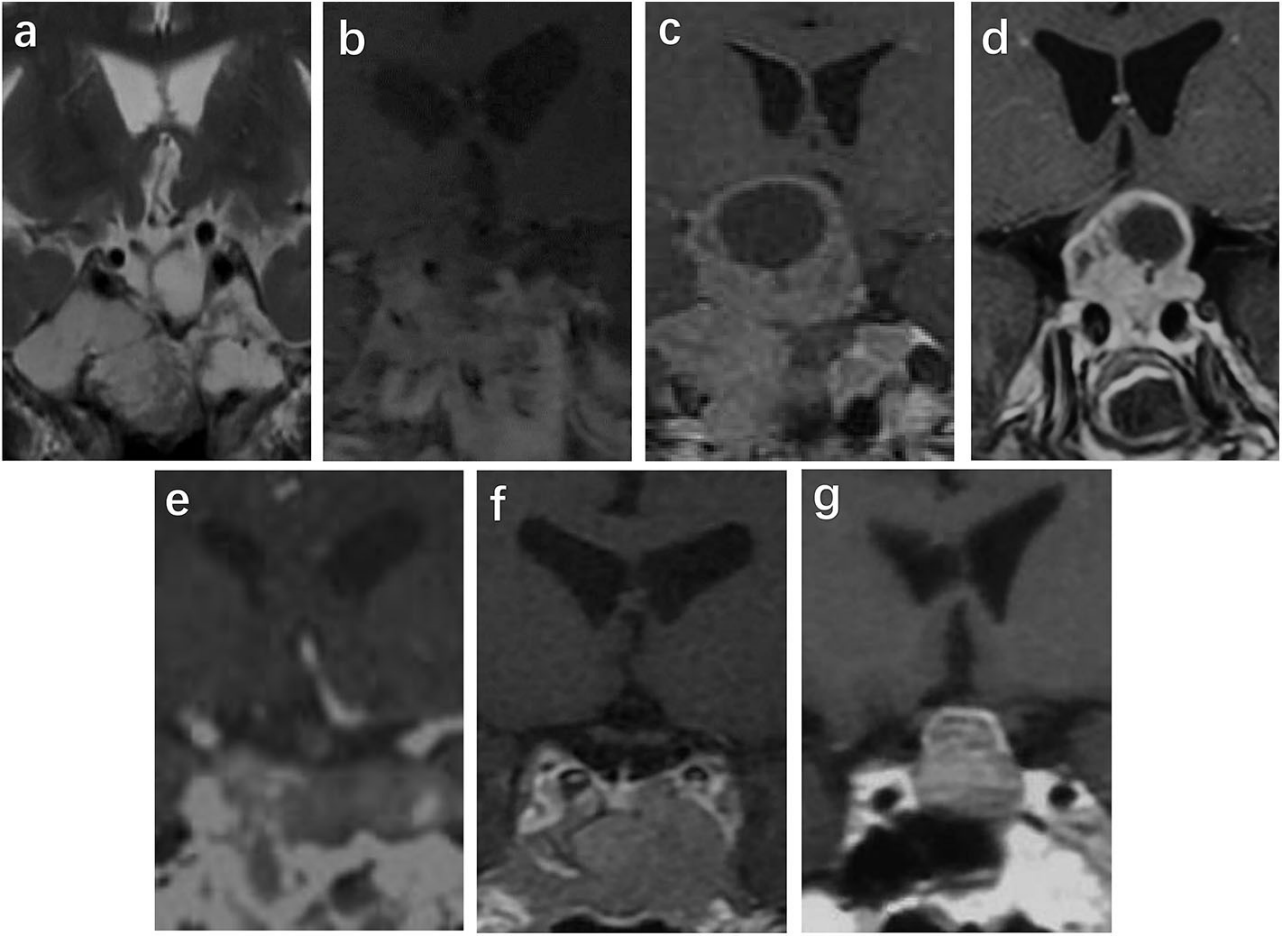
Images at the time of diagnosis of a functional SCA in cases 1–7 (a–g). Sella MRI was performed in cases 1 (a), 2 (b), 3 (c), 4 (d), 6 (f), and 7 (g). Sella CT was performed in case 5 (e) because a pacemaker was installed. There is cavernous invasion in (b), sphenoid sinus invasion in (d), and both types of invasion in (a). There are macrocystic changes and surrounding microcystic changes in (b,c). According to T2-phase MRI of case 1 (a), the tumor tended to have cystic degeneration.
